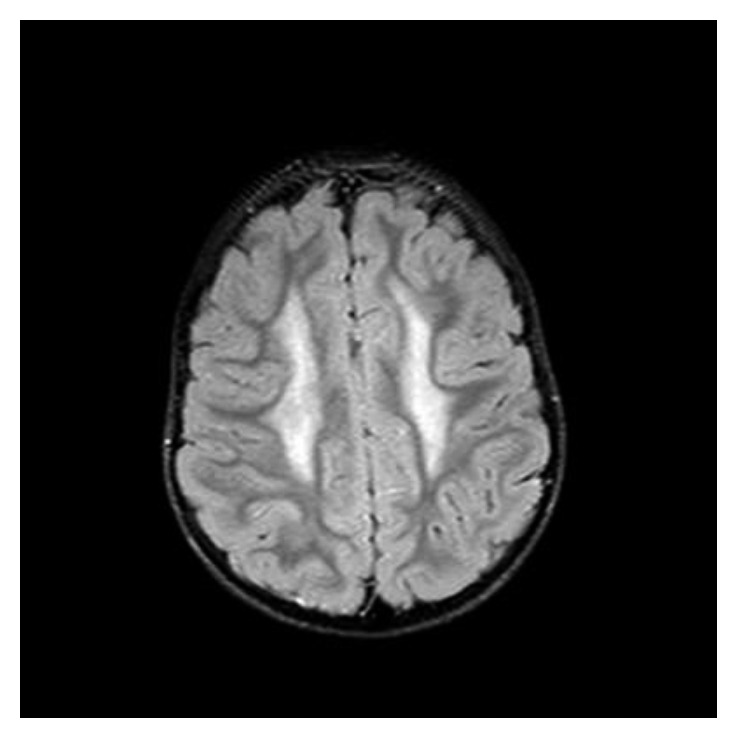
The deep white matter of the centrum semiovale bilateral demonstrates high signal intensity changes at Flair axial MR imaging.
